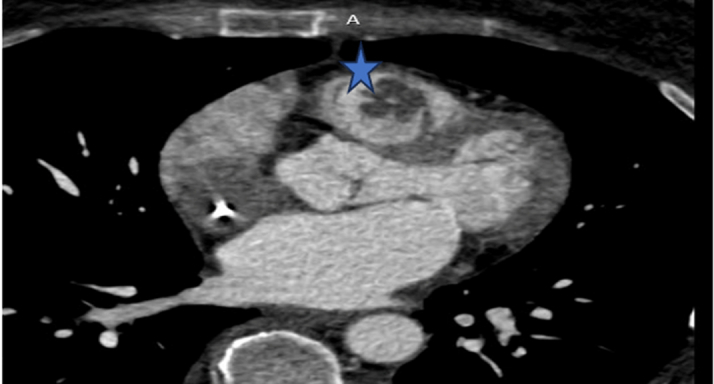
Computed Tomography Angiography Showing the Persistent Right Ventricular Mass (Star)
